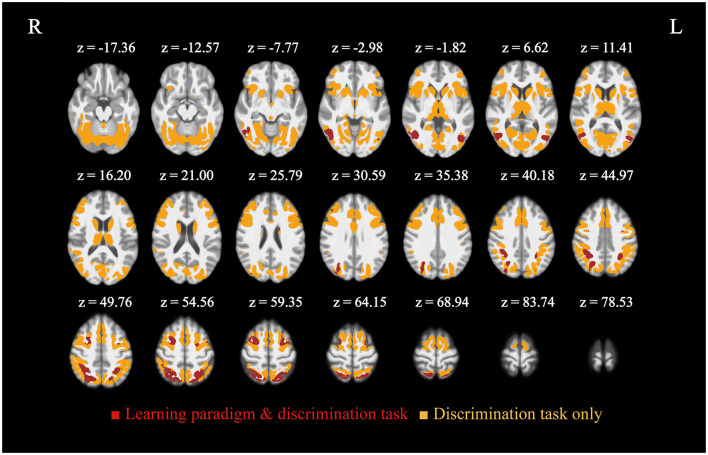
Activation elicited by the learning and attention paradigms in form of multiple comparison corrected T-maps (FDR ≤ 0.05, *n* = 34) displayed over the mean of subject T1-weighted images. Regions common to both learning and attention are shown in red, those exclusive to attention are in orange. Learning paradigm activity was wholly encompassed by that from the discrimination task, no region was observed outside of it. Significant clusters of common activity were found bilaterally, namely in V5 (middle temporal gyri), middle frontal gyri, and superior parietal lobules.
